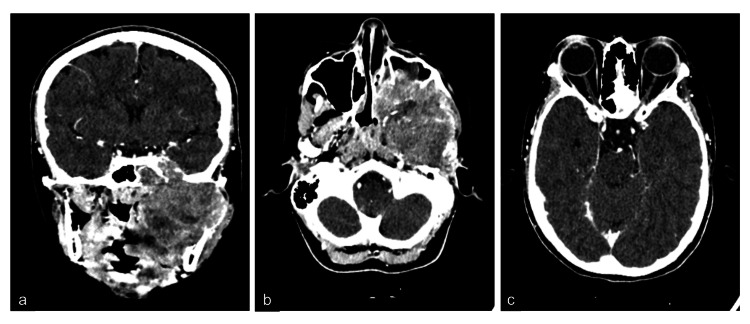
Contrast-Enhanced Computed Tomography (CECT) brain, orbit and paranasal sinus a) Coronal CT showed a significantly larger, ill-defined heterogeneous enhancing mass on the left side of the face. It occupied the left infratemporal, masticator, and parapharyngeal space with local and intracranial extension to the left temporal lobe. b) Axial CT showed bony destruction was observed at the base of the skull, lateral wall of the sphenoid, and left maxillary sinus. c) No invasion or compression of the optic nerve.
